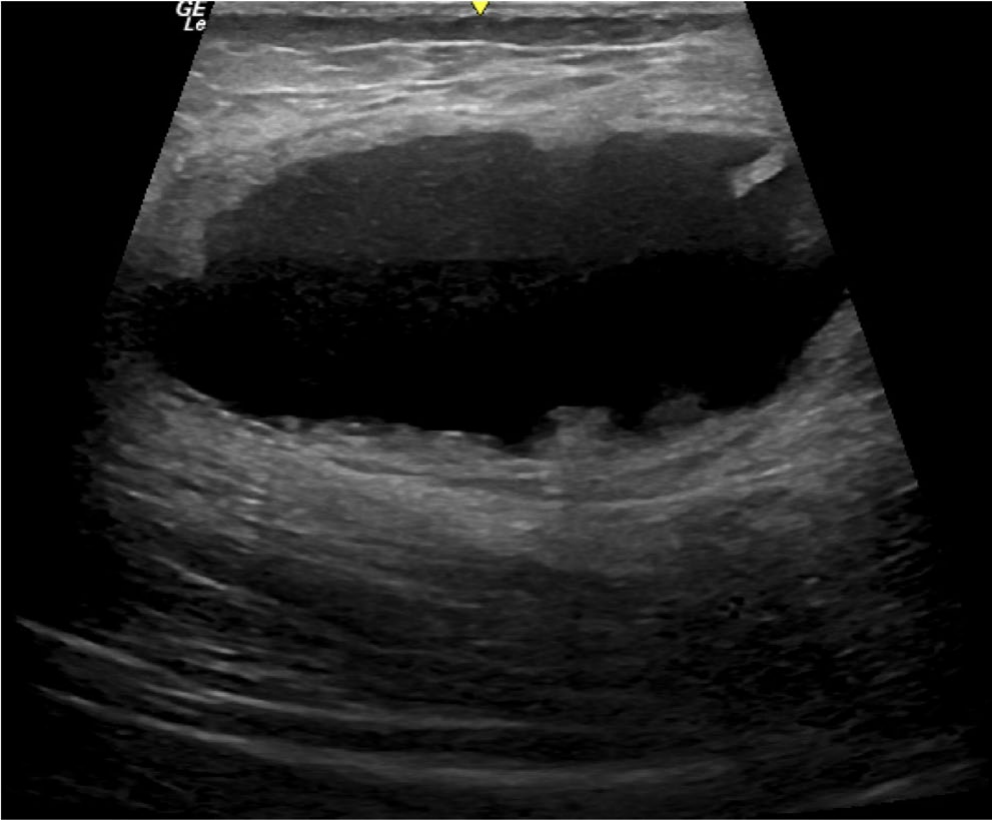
Post‐grafting fat collection. Ultrasound image of a post‐grafting fat collection with thick walls and an anechoic center, typically observed after autologous fat grafting with a complication known as central necrosis.
